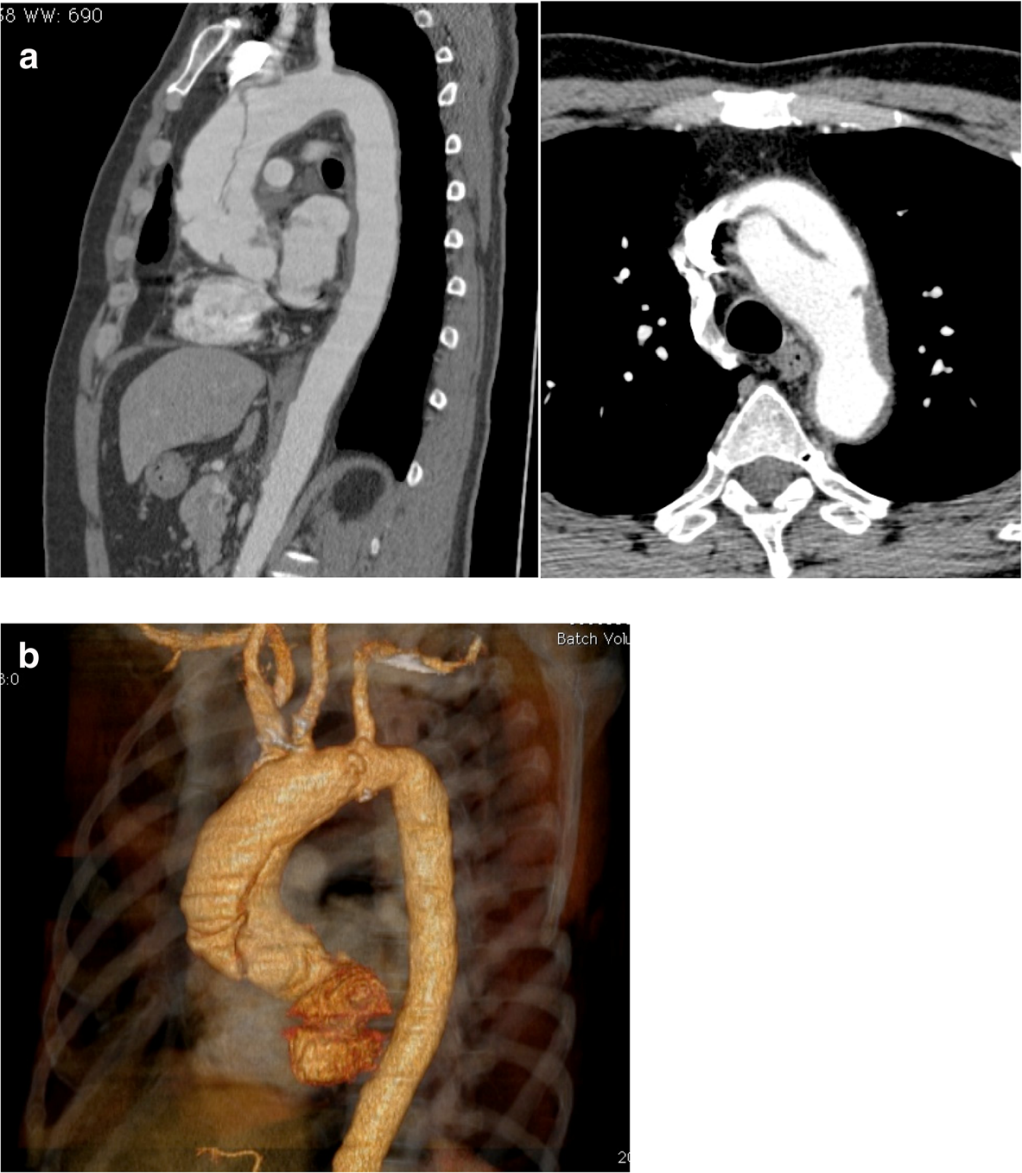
CT scan showing a Stanford type A aortic dissection. a Multiplanar reconstruction view of CT angiogram of the thoracic aorta. The maximum diameter of the ascending aorta was 51 mm. The entry was at the ascending aorta and the reentry was between the left common carotid artery and left subclavian artery. b Preoperative multi detector computed tomography
